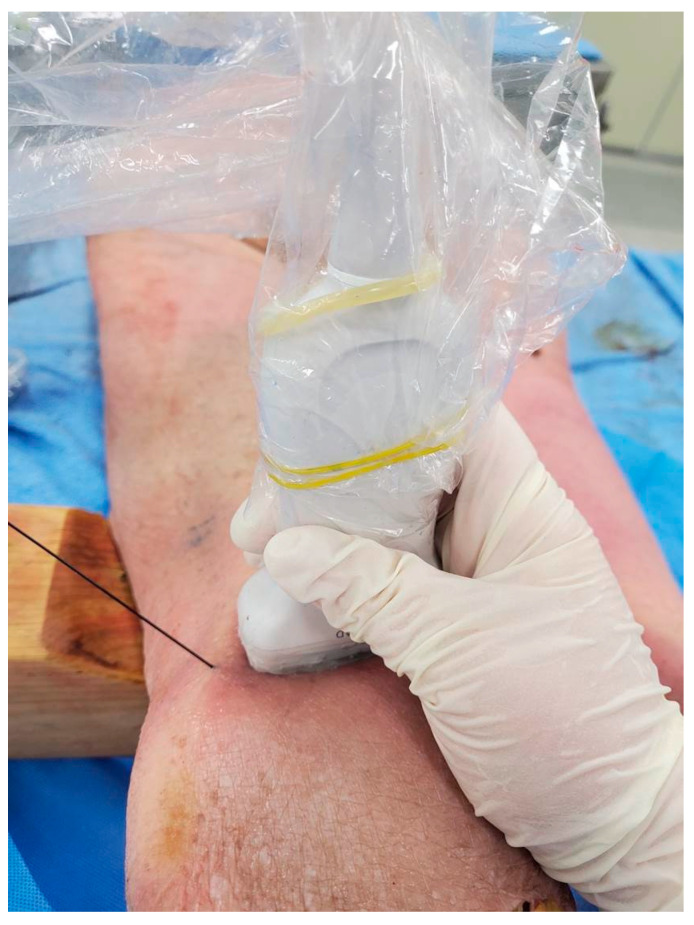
Interspace between the popliteal artery and the posterior capsule of the knee (iPACK) injection performed with a linear ultrasound probe with the cadaver in the prone position.
